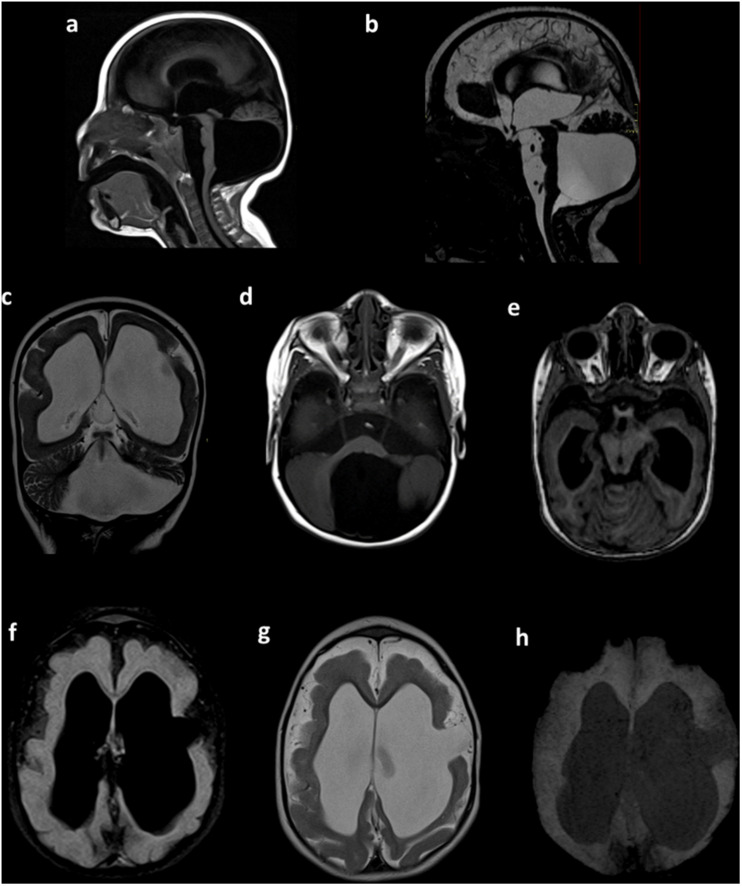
Brain MRI. Sagittal T1-weighted (a) and sagittal constructive interference in steady state (CISS) (b) sequences showing microcephaly with a giant posterior fossa cyst, a small and displacement of the brain stem, and tightening of the posterior fossa. Coronal T2-weighted images (c) showing ventricular dilatation, cortical and white matter narrowing, and posterior fossa cyst. Axial T1-weighted images (d) showing a posterior fossa cyst. Axial T1-weighted multiplanar reconstruction (e) showing a narrow posterior fossa, cerebellar vermis with hypoplasia, and superior and lateral deviation of the cerebellum. Axial FLAIR (f) and axial T2-weighted sequences (g) showing hydrocephalus and anomalous cerebral cortex with lissencephaly and left frontal open-lip schizencephaly. Axial susceptibility-weighted image (h), with the absence of intracranial calcifications. Preliminary data of this study were previously presented in the IBRO meeting 2019 (Candelo et al., 2019).
